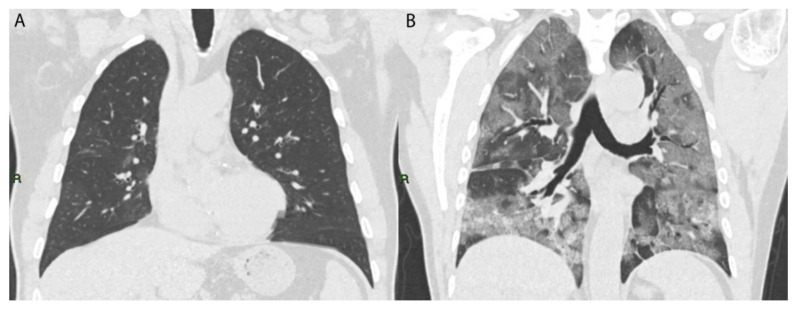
Coronal TC image of two COVID-19 patients: (A) patient with low CT score (5/25); (B) patient with high CT score (24/25).
